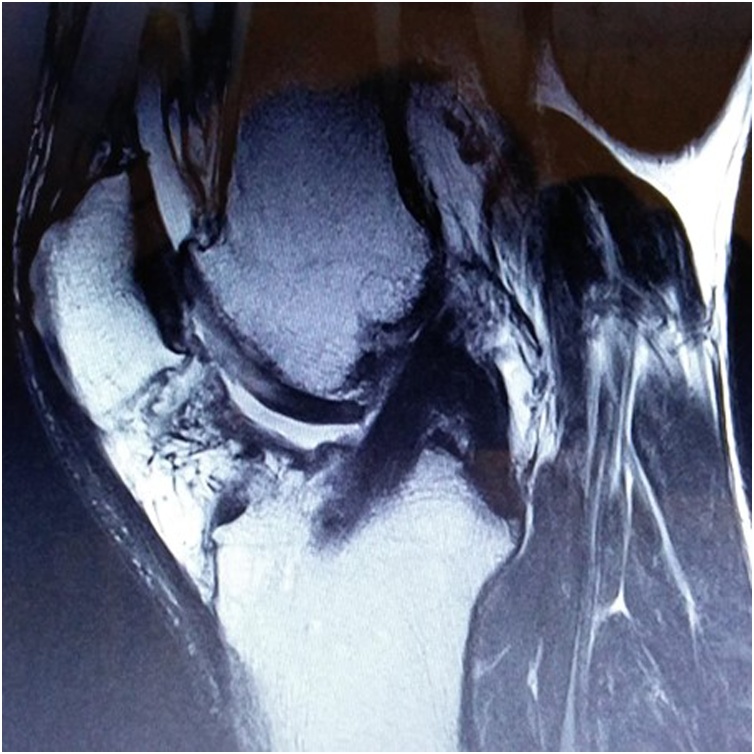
Magnetic resonance imaging conducted 4 months after surgery, showing normal graft thickness and T2-hypointense homogeneous signal, regular borders, and full graft integration in the tunnels.
